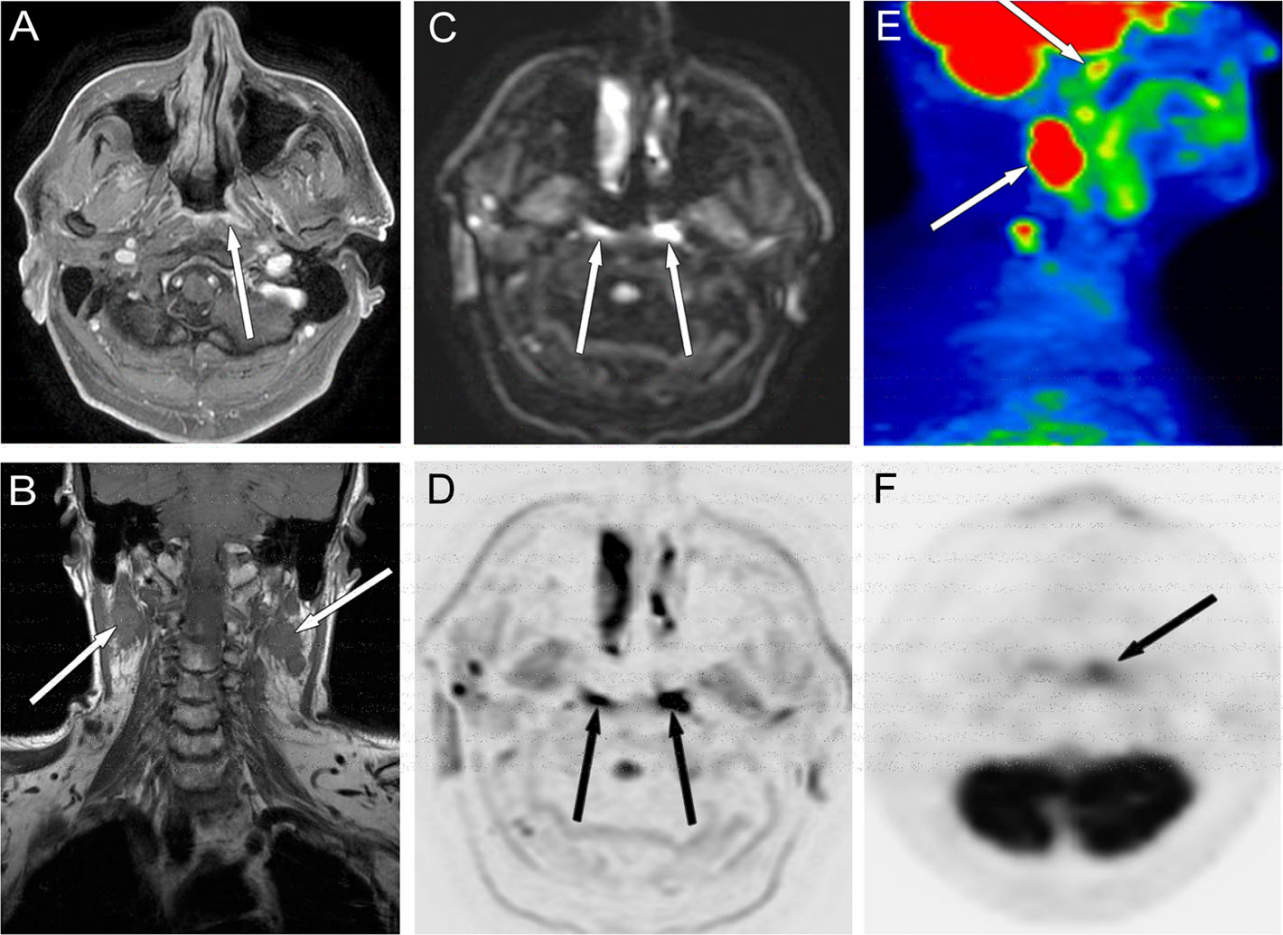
Images collected from a 64-year-old male with T1 nasopharyngeal carcinoma and bilateral metastatic nodes. Axial contrast-enhanced T1-weighted fat suppression (CET1WFS) image (a) shows a small enhancing lesion in the left side of the nasopharynx (*arrows*). Coronal T1W image (b) shows bilateral LN involvement. Bilateral diffusion restriction with signal increase can be seen in the axial trace DW images (c and d, *b*-value: 1,000 s/mm^2^, ADC: left 0.772, right 0.758 ×10^−3^mm^2^/s in the PT and 0.991 ×10^−3^mm^2^/s median ADC value was found in the LNs). Increased uptake corresponding to the occult primary tumour (*arrow*) can be seen on the sagittal PET/CT fusion image (e) of metastatic nodes (*lower arrow*) as well as on the axial FDG-PET scan (f). SCC of nasopharynx, stage T1N2M0
